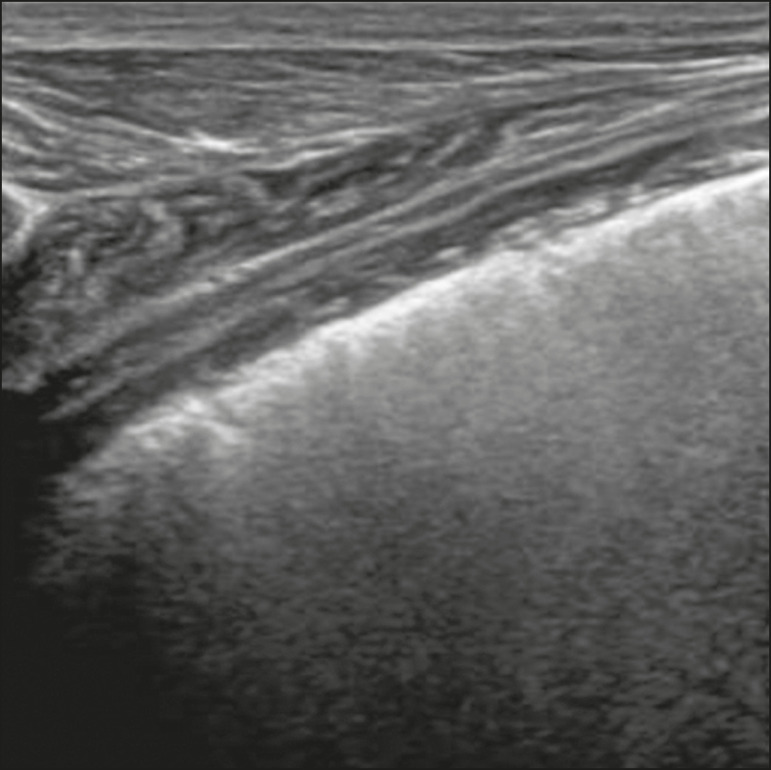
Chest ultrasound in a patient with COVID-19. Thickening and pleural irregularity, denote initial findings of the disease, can be observed using a highfrequency linear transducer.
